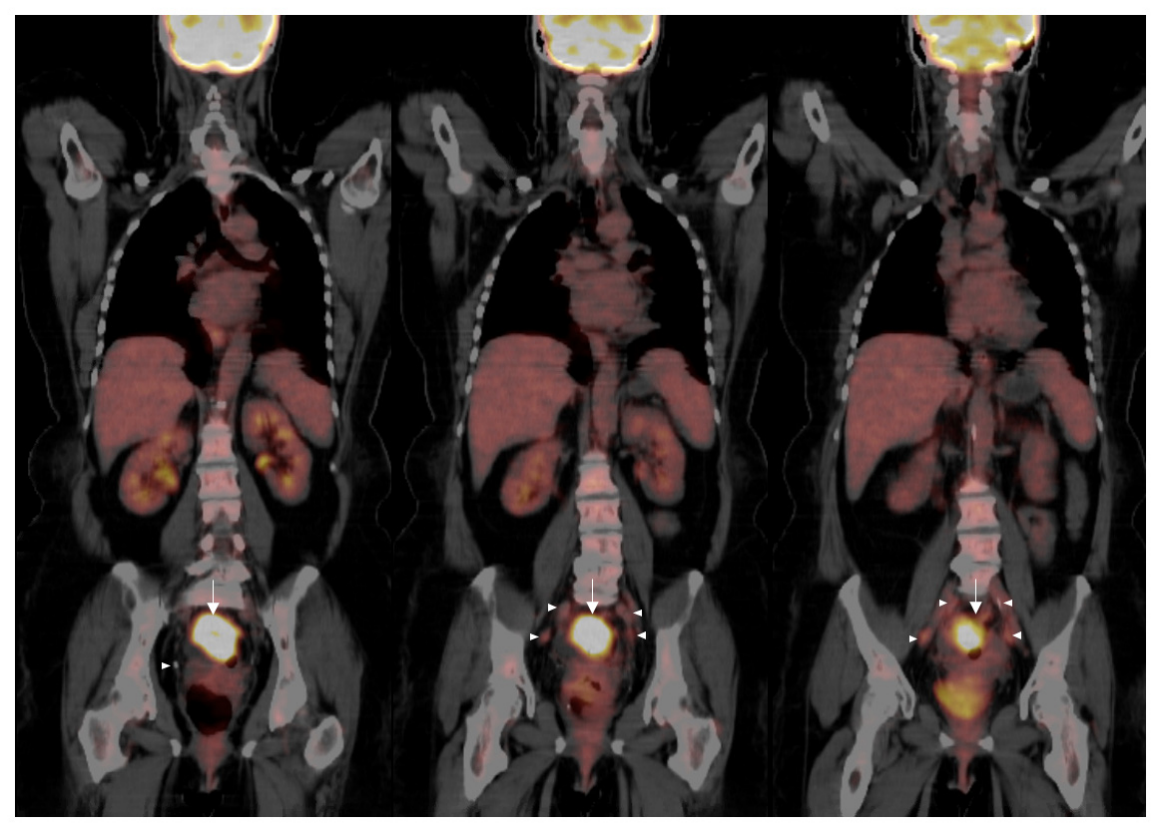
PET scans demonstrate a rectal malignancy. Imaging reveals a large focus of hypermetabolic activity in the rectosigmoid colon with a SUV of 16 and diffuse stranding in the region (
